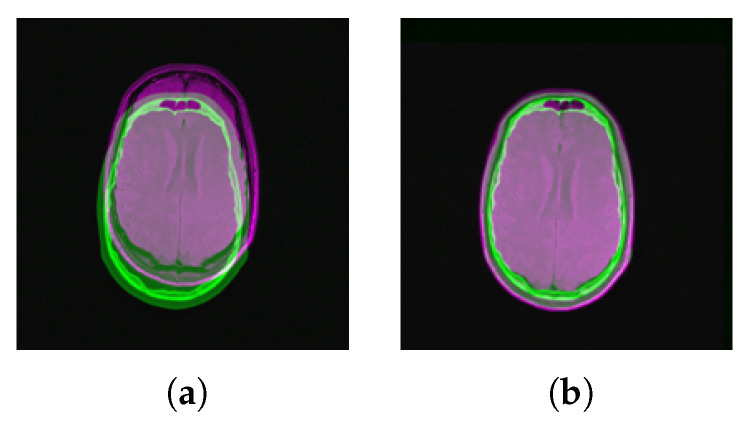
Pairwise display of a sample CT and PD-MRI scans from RIRE database. (a) Unregistered (MI = 0.8276), (b) Registered using the proposed transformation followed by mono-modal registration (MI = 1.1715).
